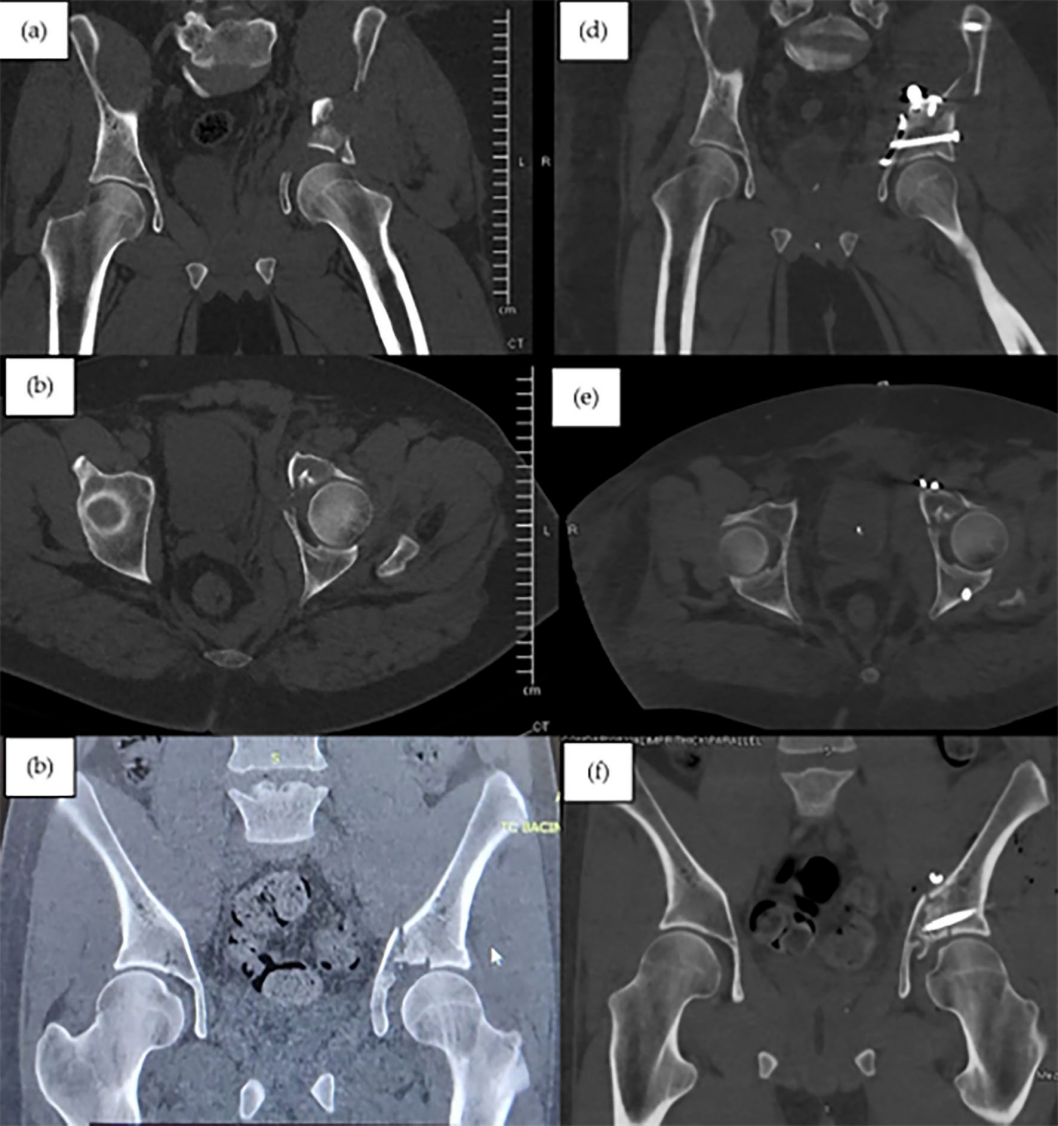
a–c Preoperative CT scan demonstrating impacted joint fragment of the acetabular roof; d–f postoperative CT scan after arthroscopic-asssited reduction and fixation, the postoperative protocol was on weight-bearing for 2 months, immediate passive assisted and active progressive range of motion of the hip
